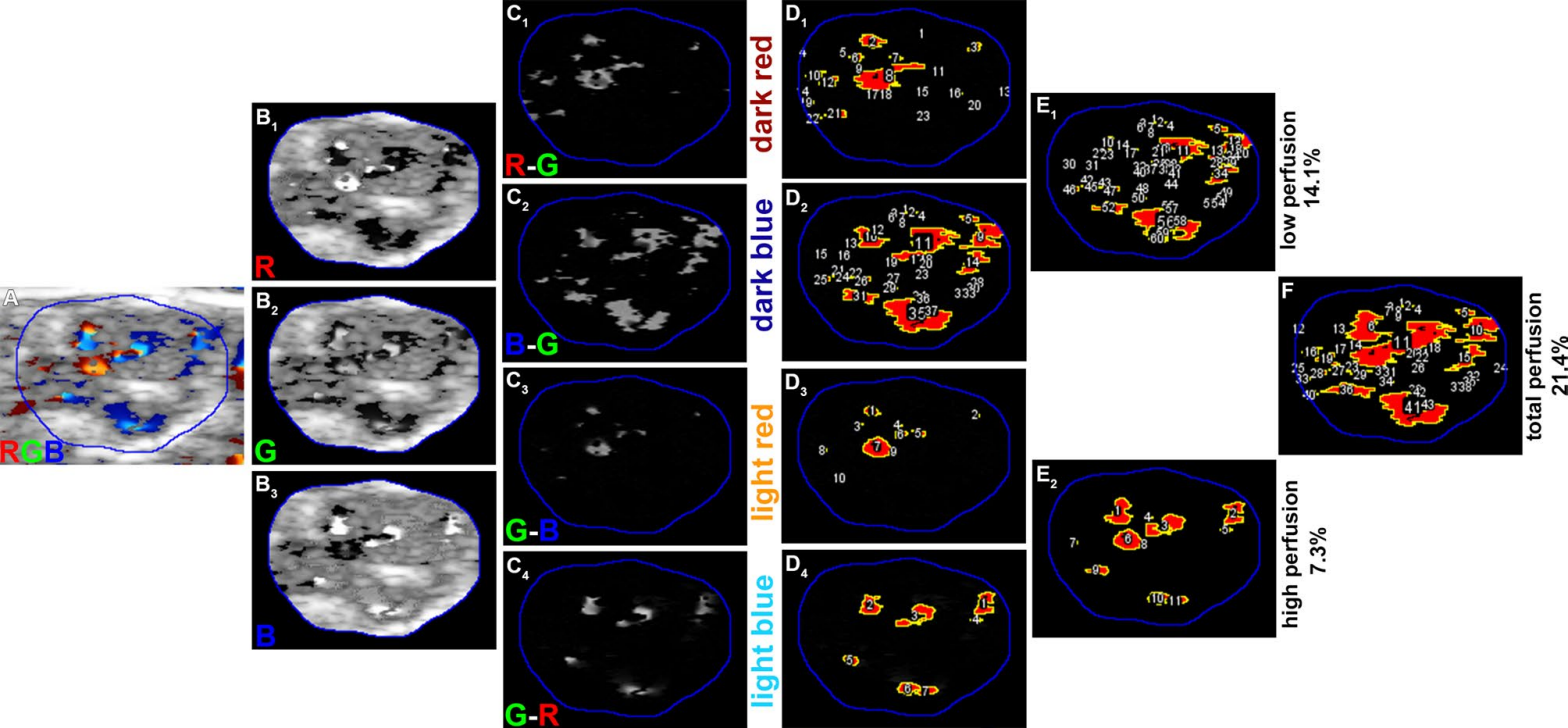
Exemplary analysis of color Doppler neurosonographs and subsequent signal selection. The color Doppler image (A) was split into the red, green and blue channel (B) and image subtraction steps were performed (C) followed by subsequent signal selection via “MaxEntropy”-thresholding and signal selection (D). Since the direction of blood flow is not analyzed, the low velocity perfusion signals (D_1_,D_2_) as well as the high velocity perfusion signals (D_3_,D_4_) were merged via maximum projections, resulting in direction-independent low velocity perfusion signals (E_1_) and high velocity perfusion signals (E_2_). For quantification of the total perfusion, these signals were merged via maximum projections respectively (F).
